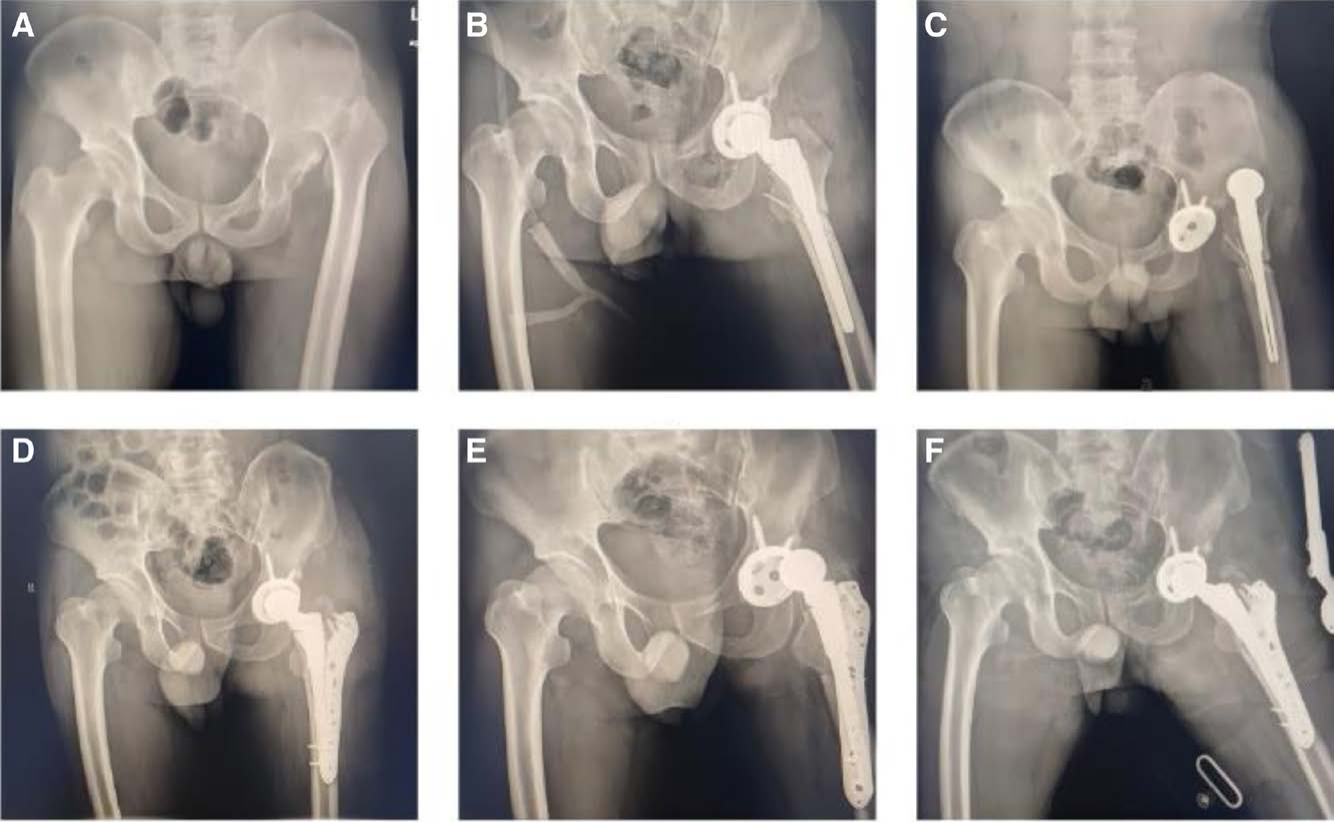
Prosthetic dislocation combined with surrounding fractures for a 48-yr-old male patient. (A) Preoperative pelvis X-ray. (B) Postoperative pelvis X-ray. (C) Anterior dislocation combined with surrounding fractures after surgery. (D) Pelvic X-ray 3 mo after revision surgery. (E) Anterior dislocation after revision surgery. (F) Pelvic X-ray after manual reduction surgery.
